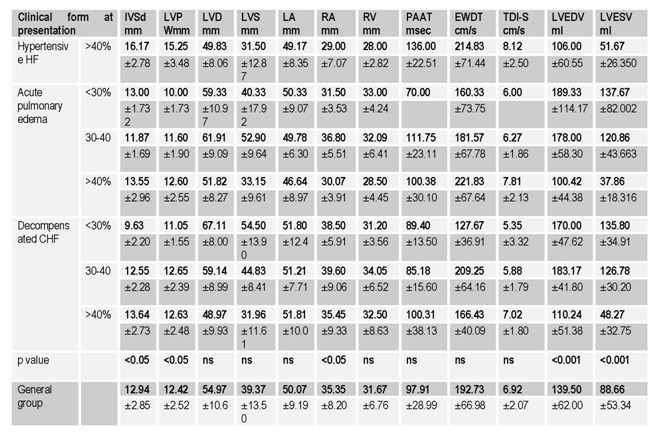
Echocardiographic characteristics according to the clinical form ar presentation and LVEF (mean±SD)
